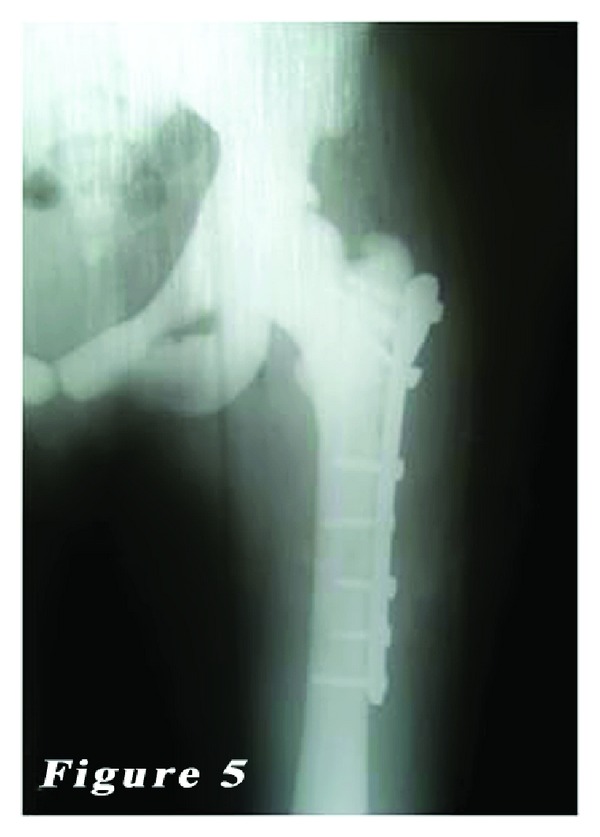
Postoperative left femur radiograph of the second patient.
